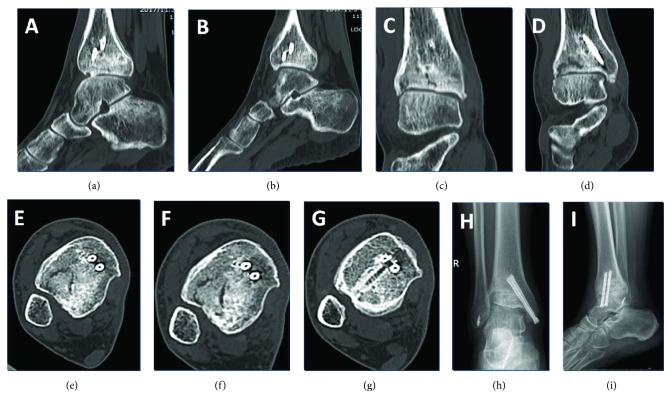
12 months posttreatment: the ankle CT scanning and anteroposterior and lateral radiographs images. (a–f) Ankle CT scanning images of the right ankle. (h) Anteroposterior radiograph of the right ankle. (i) Lateral radiograph of the right ankle.
